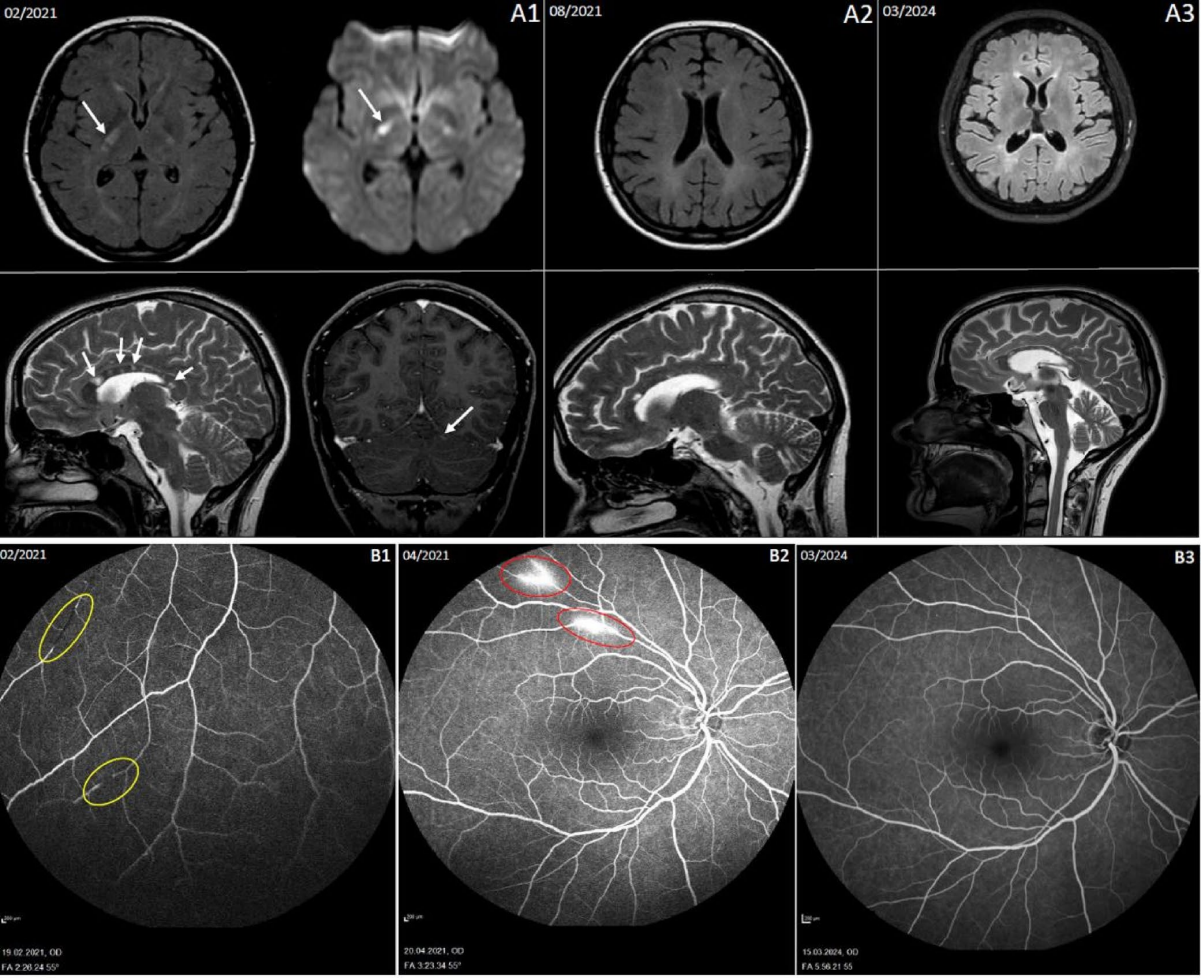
(A1) Cranial MRI scan from February 2021. Top panel: Axial fluid‐attenuated inversion recovery (FLAIR) sequence (left) and axial diffusion‐weighted imaging (DWI) (right). Bottom panel: Sagittal T2‐weighted turbo spin echo (T2/TSE) sequence (left) and coronal T1‐weighted fast field echo (T1w FFE SPIR) sequence with contrast agent (right). The imaging findings are indicative of an acute‐florid phase of a chronic inflammatory CNS disease, with a differential diagnosis of SuS syndrome, as evidenced by multiple corpus callosum lesions (white arrows, bottom panel, left) and diffusion‐restricted string‐of‐pearls‐like arranged lesions in the right posterior limb of the internal capsule (white arrows, top panel) and one cerebellar lesion (white arrows, bottom panel, right). (A2) Cranial MRI scan from August 2021. Top panel: Axial FLAIR sequence. Bottom panel: Sagittal T2/TSE sequence. Two months after initiation of natalizumab therapy, no new FLAIR lesions are observed, and the previously identified lesions remain broadly largely stable, with slight regression noted in some. No evidence of blood–brain barrier disruption or diffusion restriction is present. (A3) Cranial MRI scan from March 2024. Top panel: Axial FLAIR sequence. Bottom panel: Sagittal T2/TSE sequence. Eight months after the therapy pause (the last dose was administered in July 2023), there is no significant change compared to the previous examination from September 2023. Stable residual lesions are seen in the corpus callosum and periventricular regions, with no newly demarcated lesions detected. (B1) and (B2) Fluorescein angiography from February 2021 and April 2021 (prior to initiation of Natalizumab therapy): Vascular occlusions (yellow circles), ruptures, filling defects, and vasculitic enhancements in the optic nerve, with areas of leakage (red circles). (B3) Fluorescein angiography from March 2024 (following the last dose of natalizumab in July 2023): No evidence of inflammatory activity observed.
